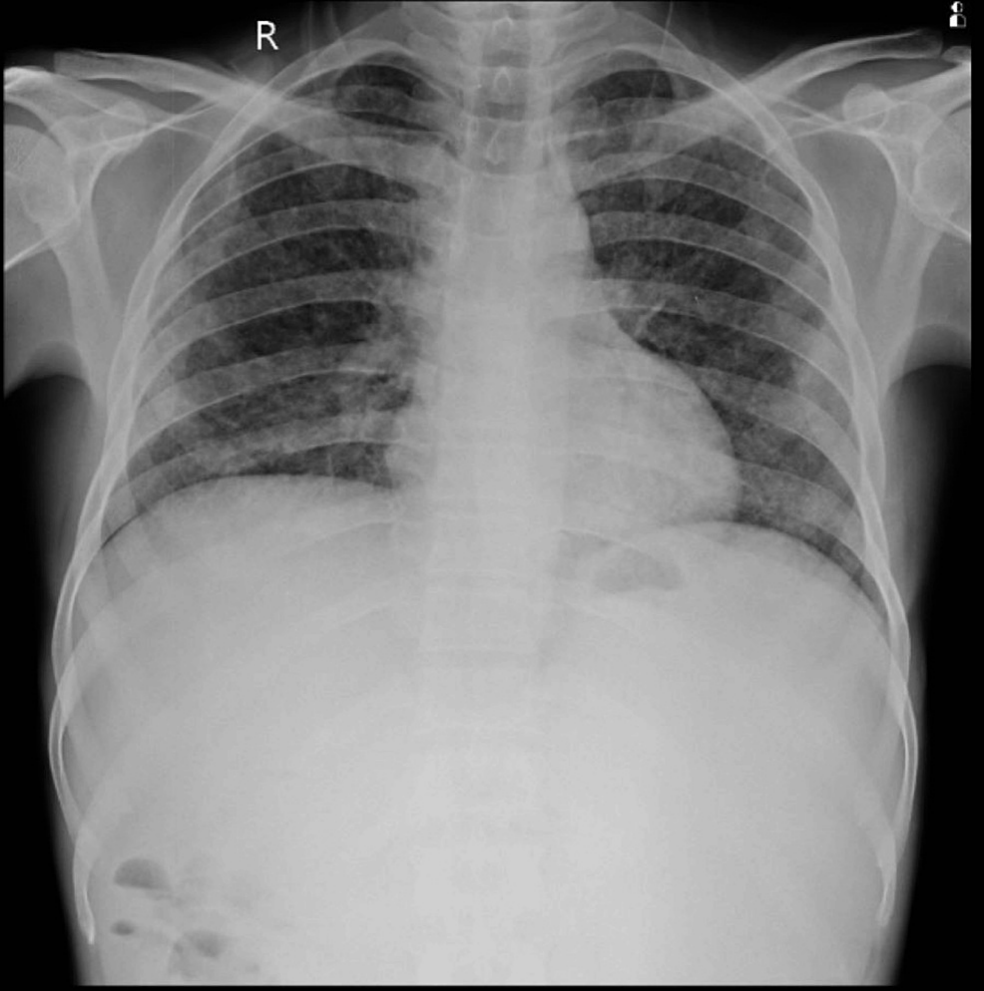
Chest X-ray of the patient showing radiological improvement
